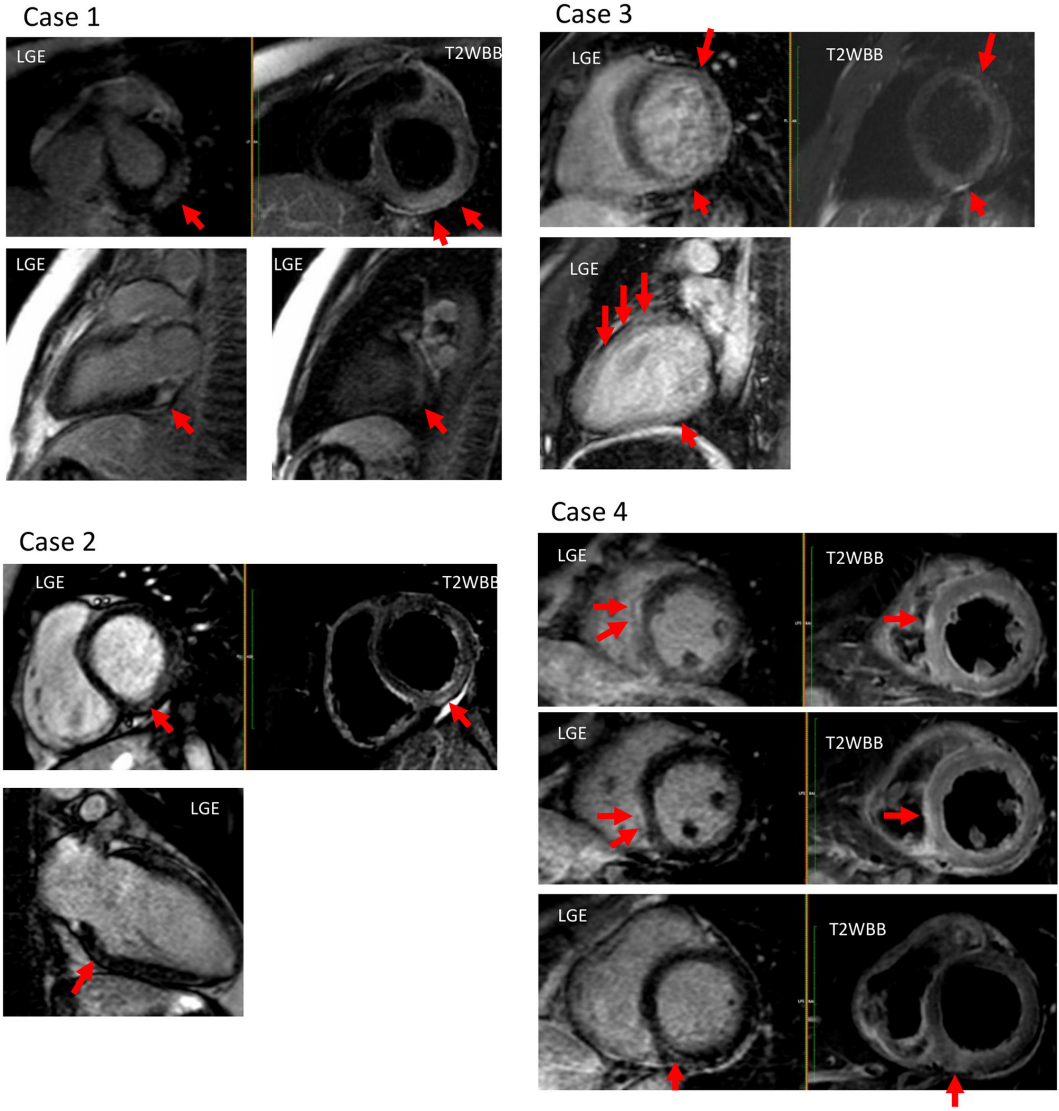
Cardiac magnetic resonance imaging (MRI) of all profiled cases. Case 1: T2 high signal intensity and late-gadolinium enhancement (LGE) were observed at the sub-epicardial wall of the basal-mid inferolateral left ventricular (LV). Case 2: T2 high signal intensity and LGE were observed at the mid wall of the basal inferior LV. Case 3: T2 high signal intensity and LGE were observed at the mid wall of the anterior, and at the inferior LV. Case 4: T2 high signal intensity and LGE were observed at the mid-wall of basal inferior, and at the sub-epicardial wall of the mid- and inferoseptum LV.
